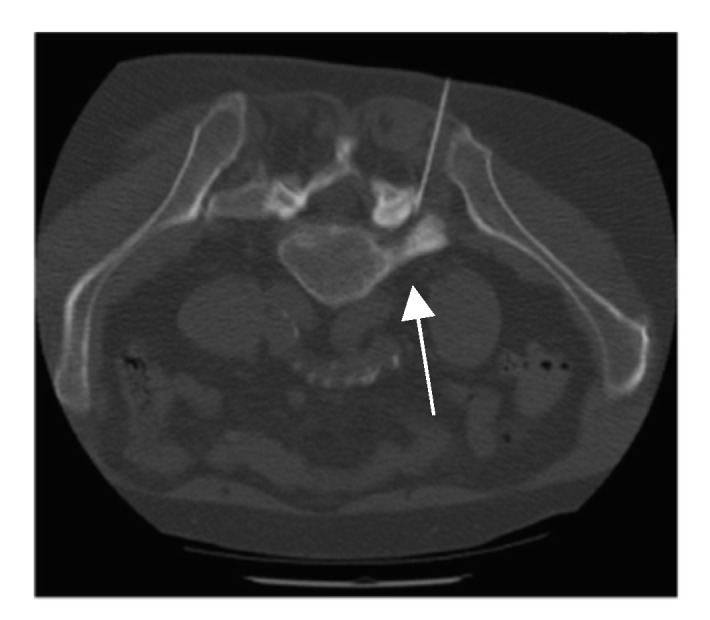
Computed tomography showing the positioning of the spinal needle lateral to the midline at the level of the affected nerve root via a transforaminal approach at the L5/S1 neuroforamen (white arrow).
